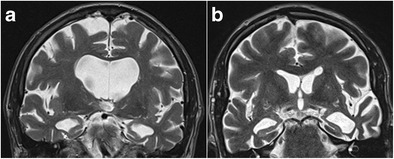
Alzheimer’s disease (a) and frontotemporal dementia (b). 3-T Coronal T2-weighted images in an 84-year-old patient with clinically advanced sporadic Alzheimer dementia show pronounced mesial temporal atrophy corresponding to an MTA score of 4 (a). In comparison, coronal T2-weighted images in a 58-year-old patient with a semantic variant of frontotemporal dementia also shows pronounced bilateral mesiotemporal atrophy (MTA score 4), along with asymmetric pronounced cortical atrophy of the left temporal neocortex (b)
